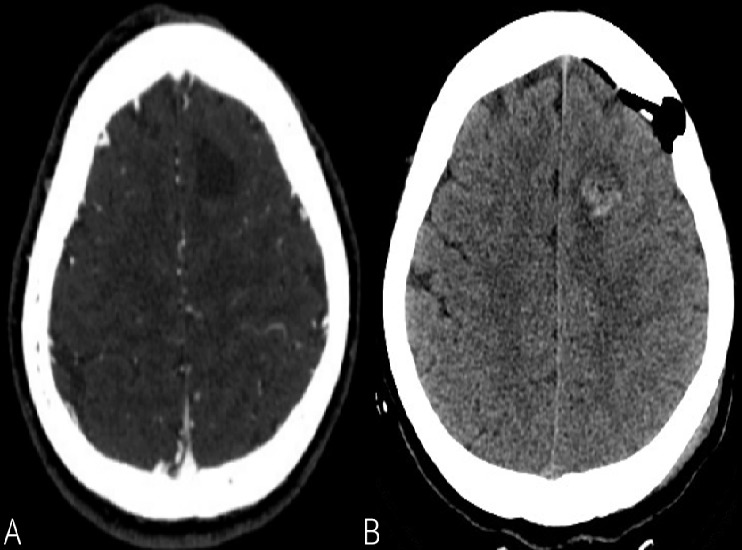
(A) angio CT axial cuts does not show any vascular malformation; (B) post-surgical CT showing cyst was removed completely
